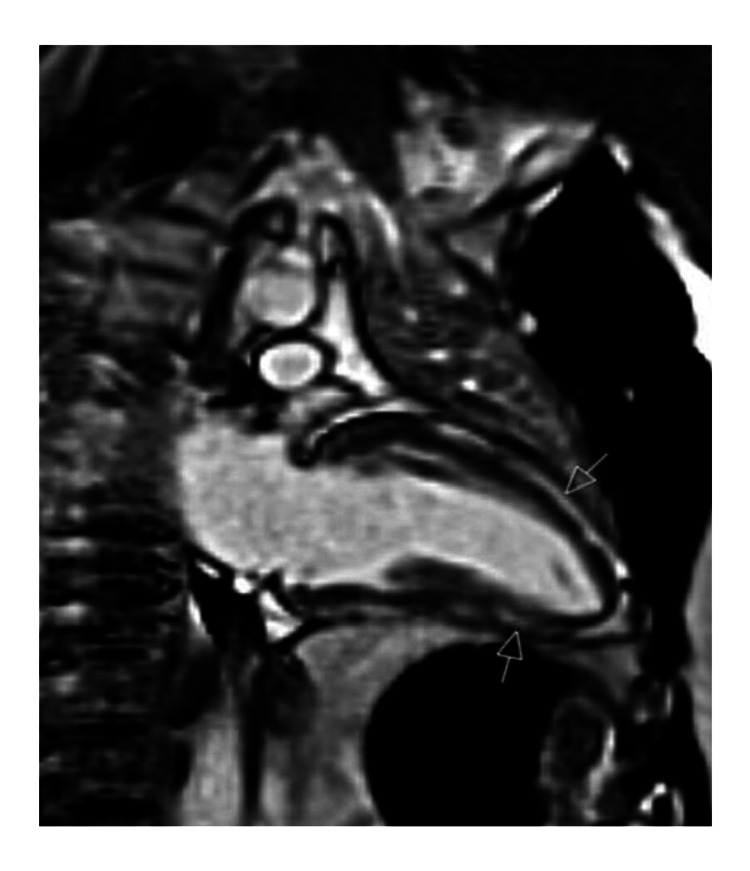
Cardiovascular magnetic resonance imaging of two-chamber view showing extensive, crescentic-shaped, subepicardial late gadolinium enhancement as shown by arrows.
